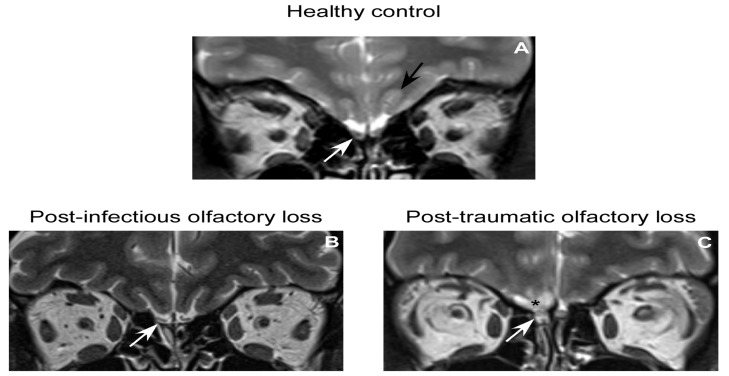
Coronal T2-weighted images of the olfactory bulbs (OBs) in normal subject (A) and in patients suffering from post-infectious (B) and post-traumatic (C) olfactory loss.
